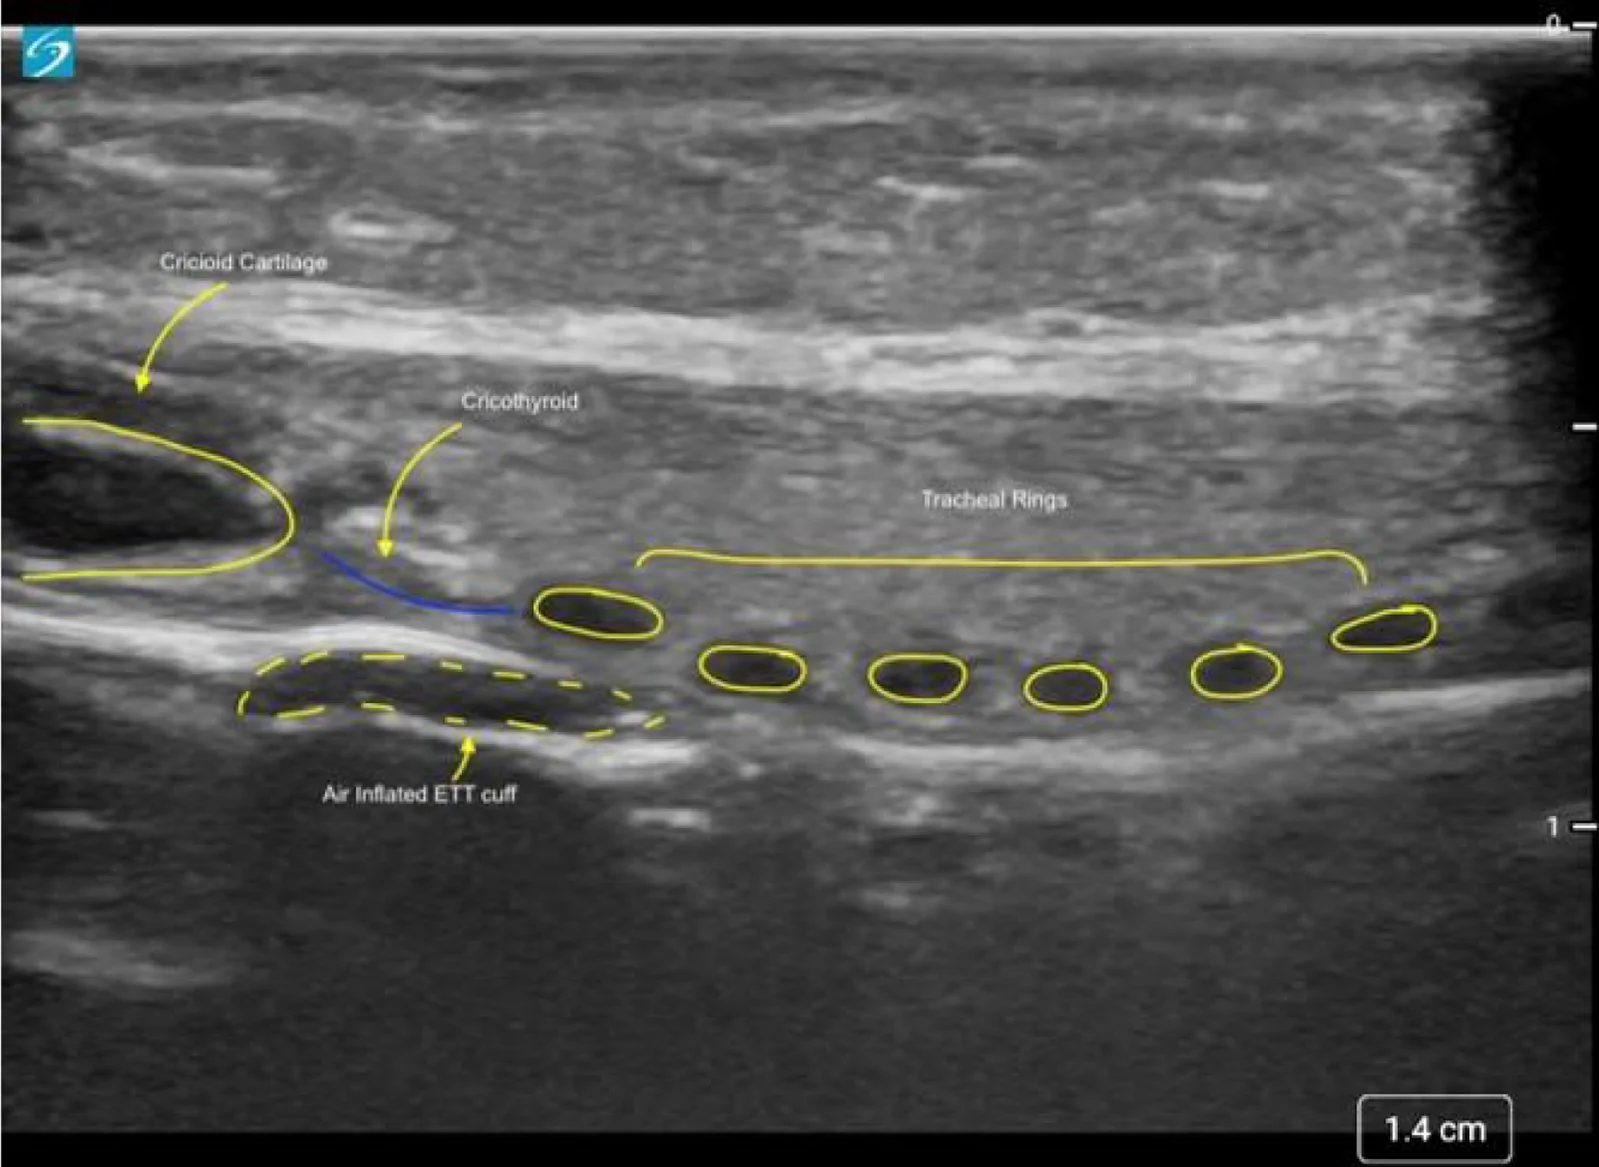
Sagittal ultrasound view of the airway showing the cricoid cartilage to the left at the top of the airway, the cricothyroid membrane (blue line), and tracheal rings. Air can be seen in the inflated endotracheal tube (ETT) cuff.
